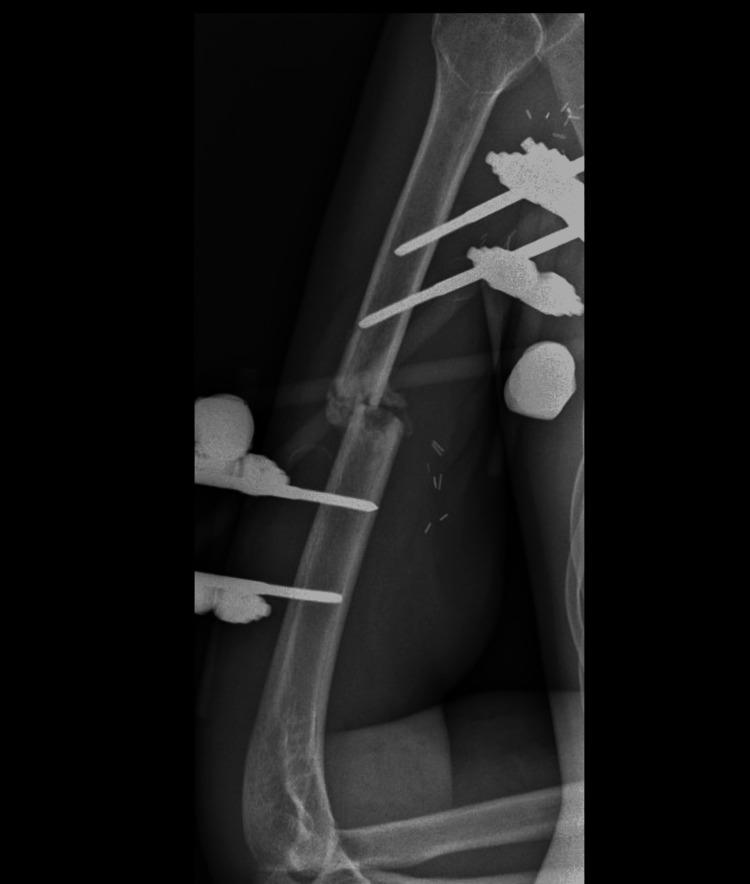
The three-month postoperative right humerus X-ray demonstrating external fixator application
